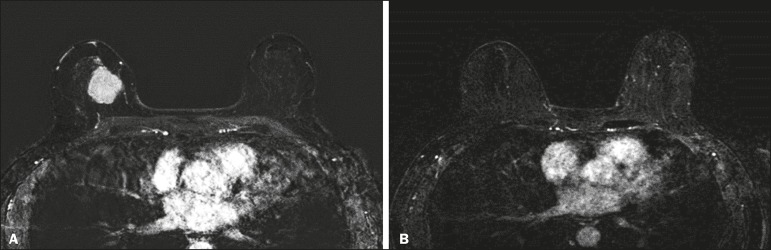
Example of a true-positive result. Pre- and post-NAC MRI (A
